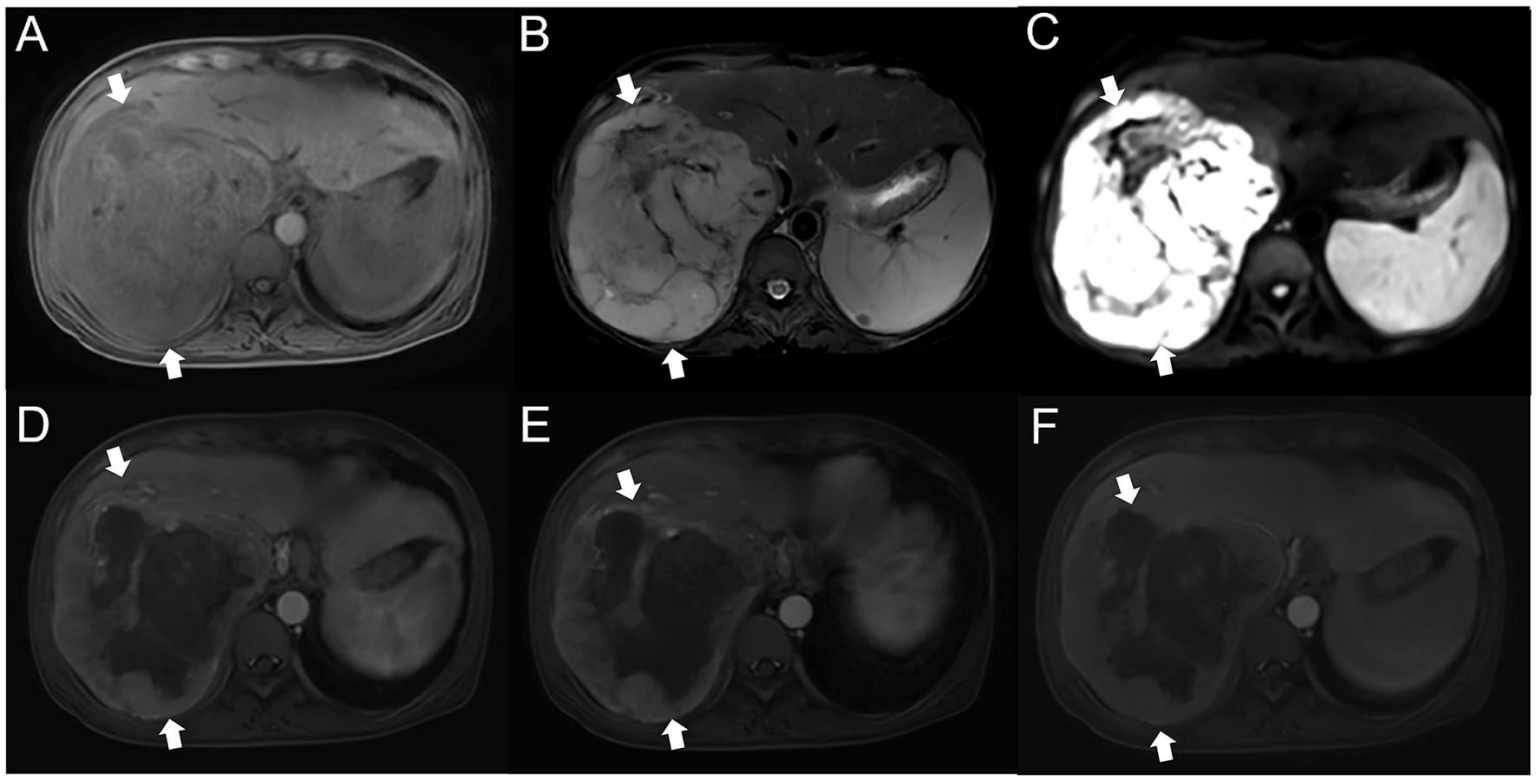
MRI of the patient's liver (A). T1WI showed the presence of clumpy and other low-confounding signal shadows in the right lobe of the liver (B). Most of the lesion site on T2WI had slightly higher signal shadows, with irregular bars of low signal shadows inside (C). On DWI, the lesion site was unevenly hyperintense (D). Contrast-enhanced MRI showed that the edge of the arterial lesion was strengthened, with significant punctate strengthening, and large areas of low intensity were seen in the lesion (E, F). The lesion site in the portal phase and delayed phase had a clear marginal contour and mild internal delayed intensification. MRI, magnetic resonance imaging; T1WI, T1 weighted image; T2WI, T2 weighted image; DWI, diffusion weighted imaging.
